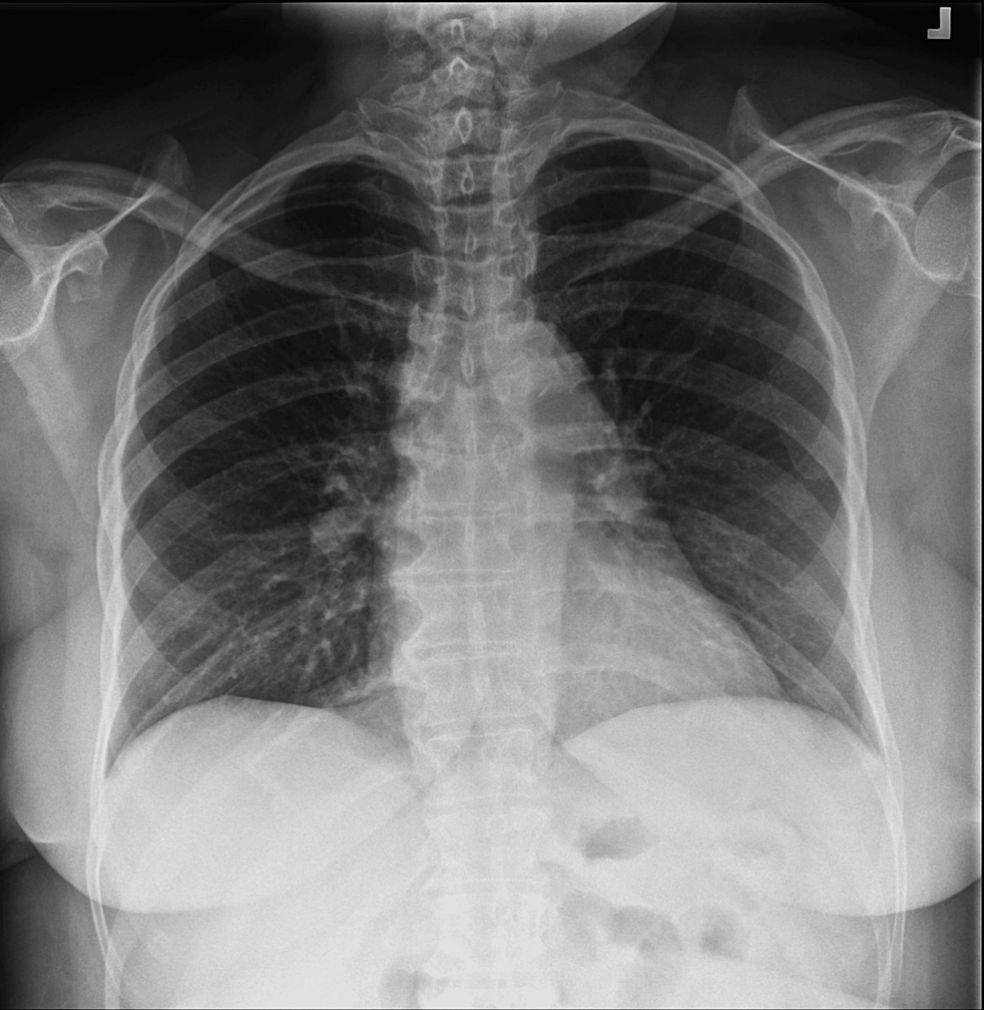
A chest X-ray revealed clear lungs with sharp costophrenic angles, but mild apical pleural thickening was noted.
